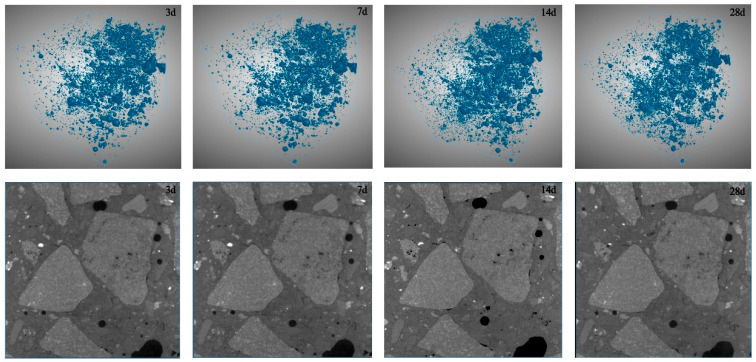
X-CT Image of SCC with 2% CAI dosage.
